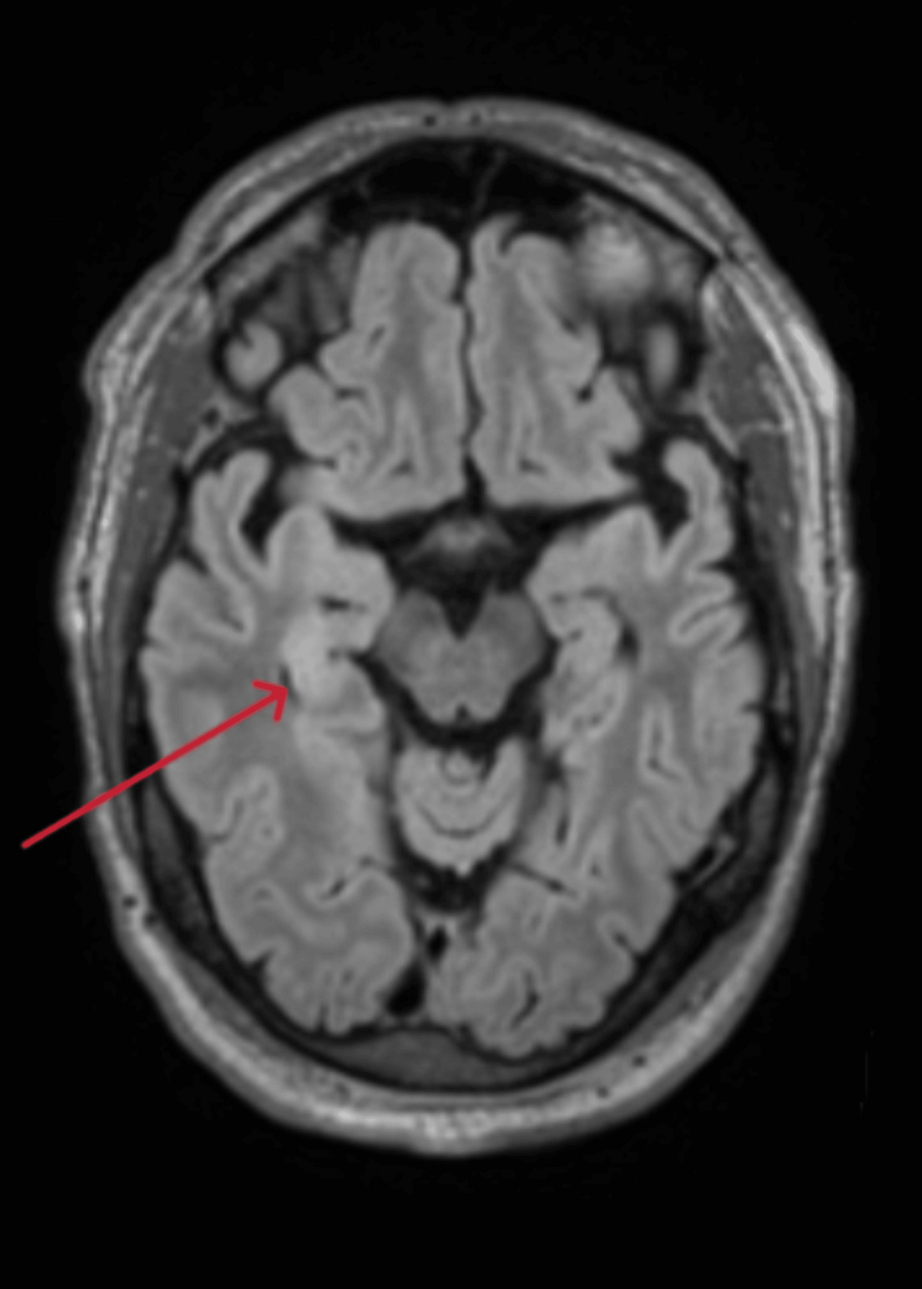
Axial brain MRI showing increased FLAIR signal in the right hippocampus MRI: Magnetic resonance imaging; FLAIR: fluid-attenuated inversion recovery
